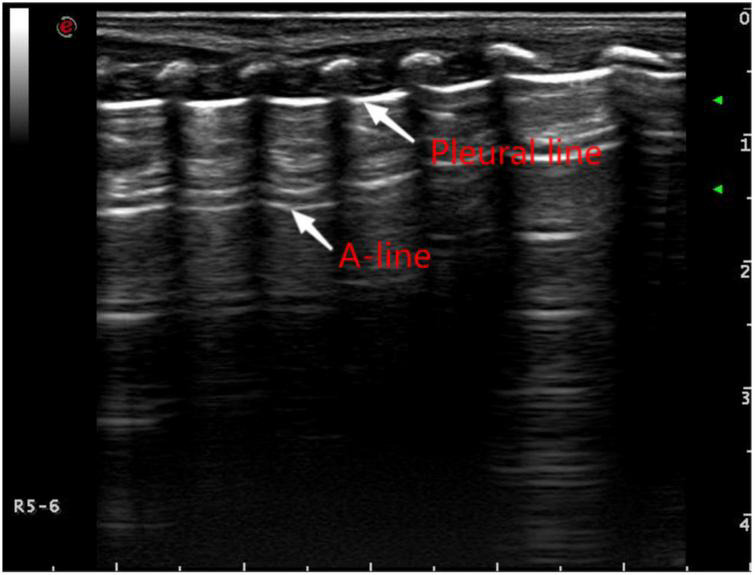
Pulmonary ultrasound findings of normal newborns. Pulmonary ultrasound shows that the pleural line and A-line are clear, smooth, and parallel like “bamboo knots.”
